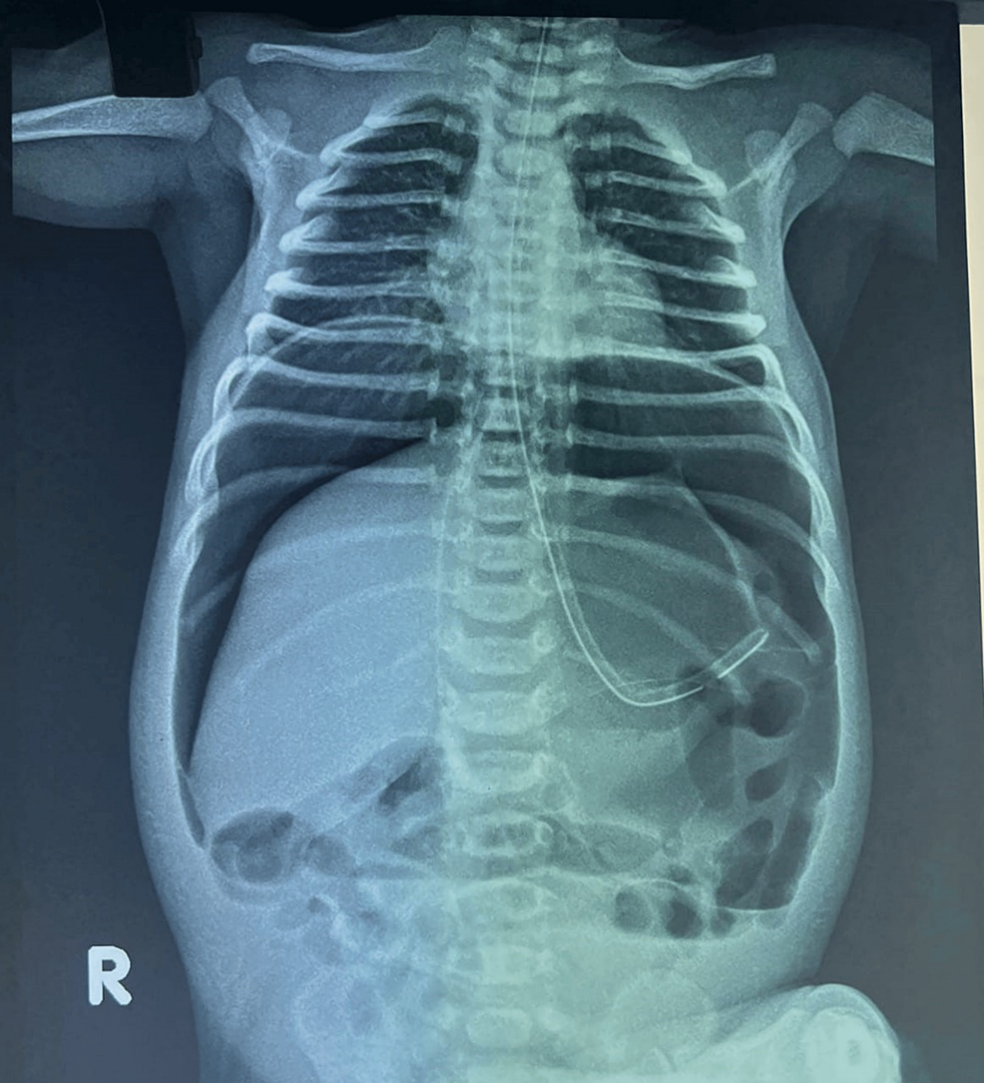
Erect abdominal X-ray of case 1 The image shows huge free gas under both domes of the diaphragm, known as the “continuous diaphragm sign,” and also shows the “falciform ligament sign” and “football sign.”
